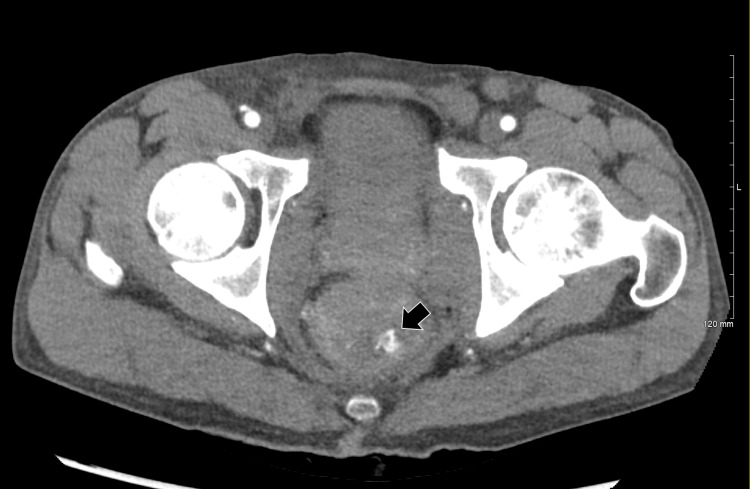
CT image in the axial plane following intravenous administration of iodinated contrast material reveals extravasation (arrow) in the lumen of the sigmoid colon.
